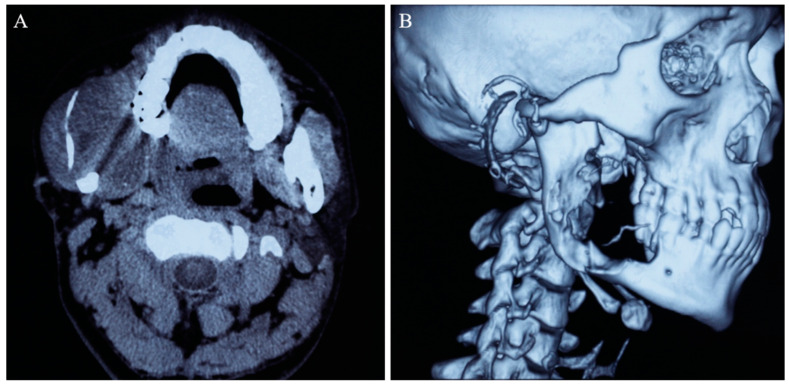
CBCT imaging (A) and three-dimensional reconstruction of the skull (B) demonstrated extensive bony destruction and soft tissue involvement by the right mandibular tumor.
